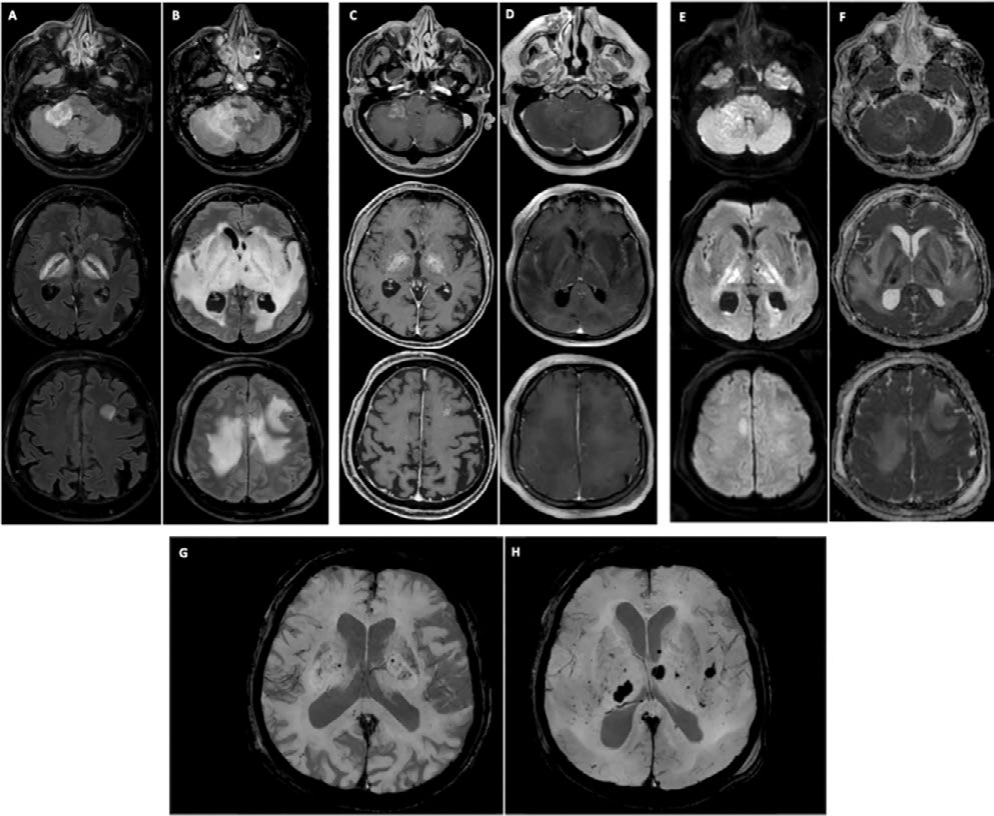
FIGURE 1 Brain MRI of the patients at ER arrival and at follow‐up five days later.
